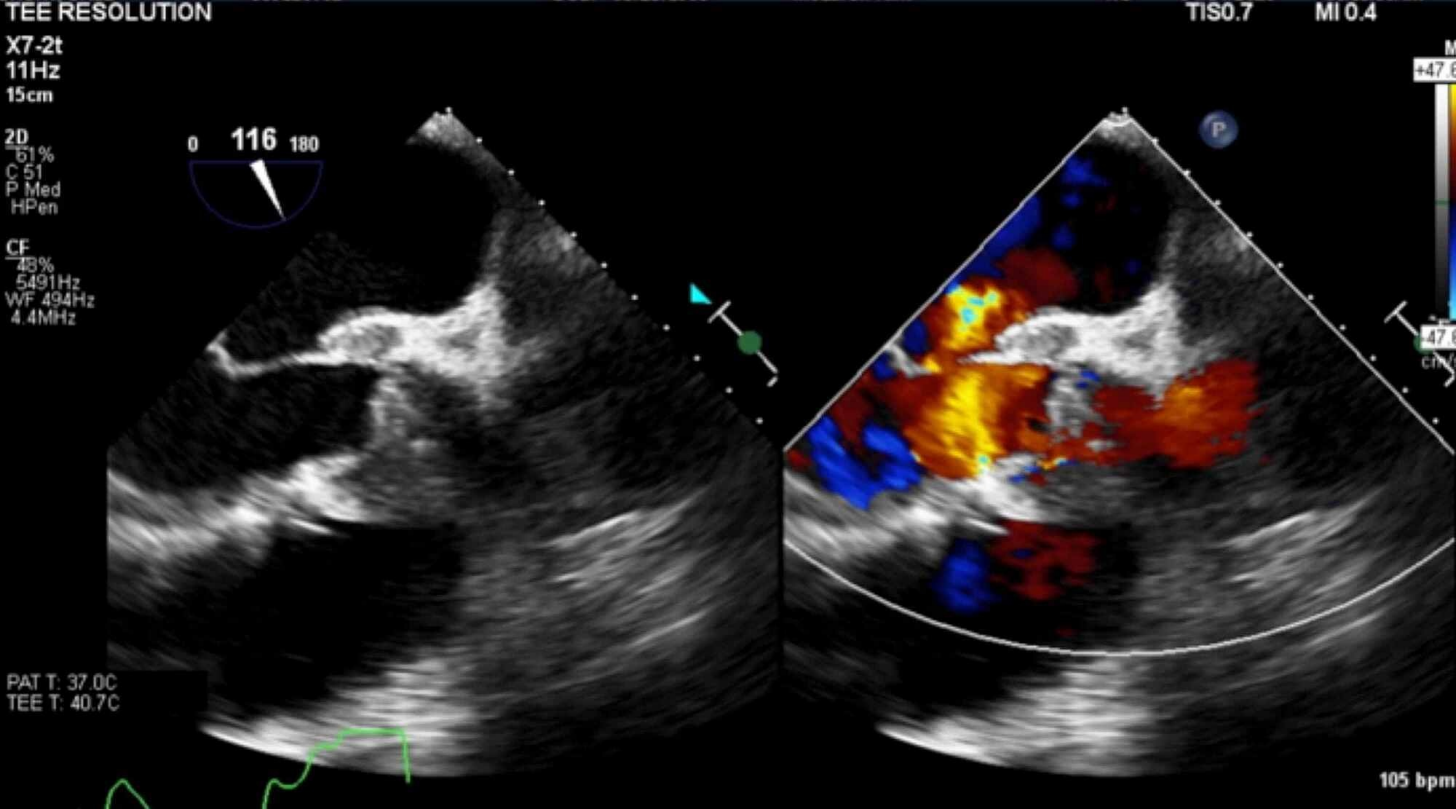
Transesophageal echocardiogram doppler showing severe aortic regurgitation (AR)
